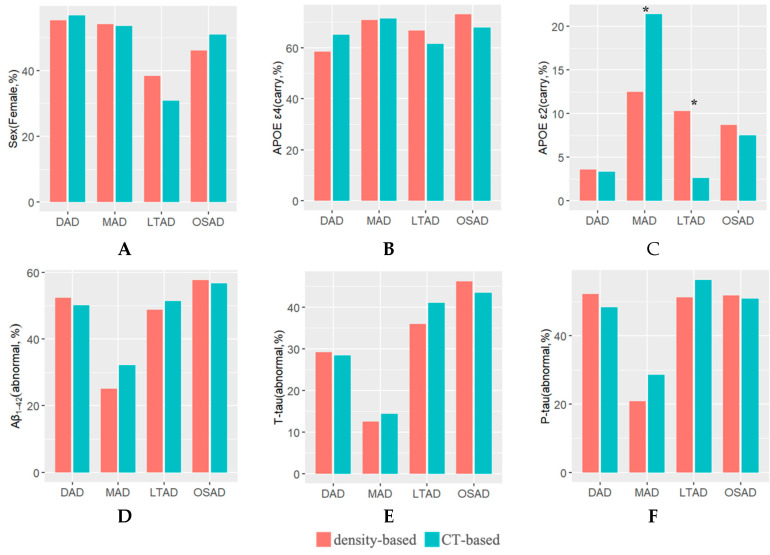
The characteristics of qualitative variables were captured by density features and CT features. The chi-square test was used for comparison between matched subtypes. *: *p* < 0.05 within the matched subtype. (A–C) The histograms for sex and APOE demonstrate what percentage of AD subjects in each subtype were females and APOE carriers, respectively. (D–F) The histograms for CSF (Aβ_1-42_, T-tau, and P-tau) indicate the abnormal percentage of AD subjects in each subtype. Abbreviations: AD: Alzheimer’s disease, APOE: apolipoprotein, Aβ_1-42_: beta-amyloid 1-42, P-tau: Phosphorylated tau, T-tau: Total tau. DAD: diffuse atrophy AD subtype, MAD: minimal atrophy AD subtype, LTAD: left temporal dominant atrophy AD subtype, MAD: minimal atrophy AD subtype, OSAD: occipital sparing AD subtype.
